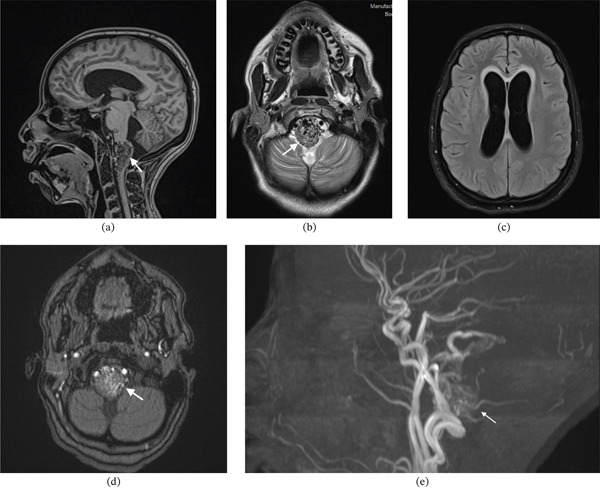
MRI of the brain demonstrating the AVM. (a) Sagittal T1‐weighted image shows multiple serpiginous flow voids at the cervicomedullary junction with focal expansion and anterior displacement of the medulla. (b) Axial T2‐weighted image also shows the abnormal flow voids. (c) Axial FLAIR image demonstrates acute hydrocephalus with ventricular enlargement. (d) Axial postcontrast T1‐weighted image confirms an enhancing vascular nidus. (e) 3D time‐of‐flight MR angiogram delineates the nidus and its arterial feeders.
